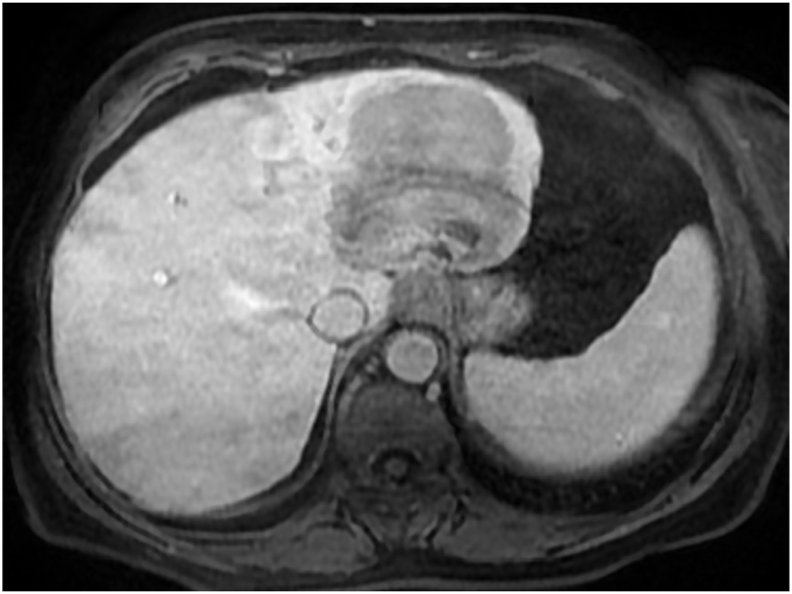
A magnetic resonance imaging showing a voluminous tissue mass of the left liver.
